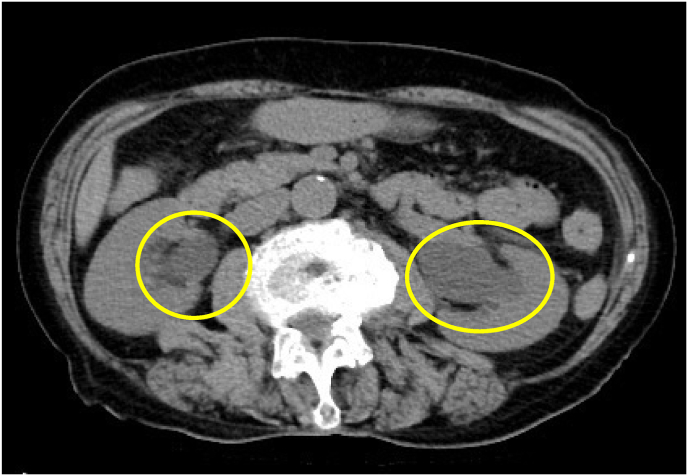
Axial section of CT. Bilateral hydronephrosis is observed on computed tomography (yellow circles). (For interpretation of the references to colour in this figure legend, the reader is referred to the Web version of this article.)
